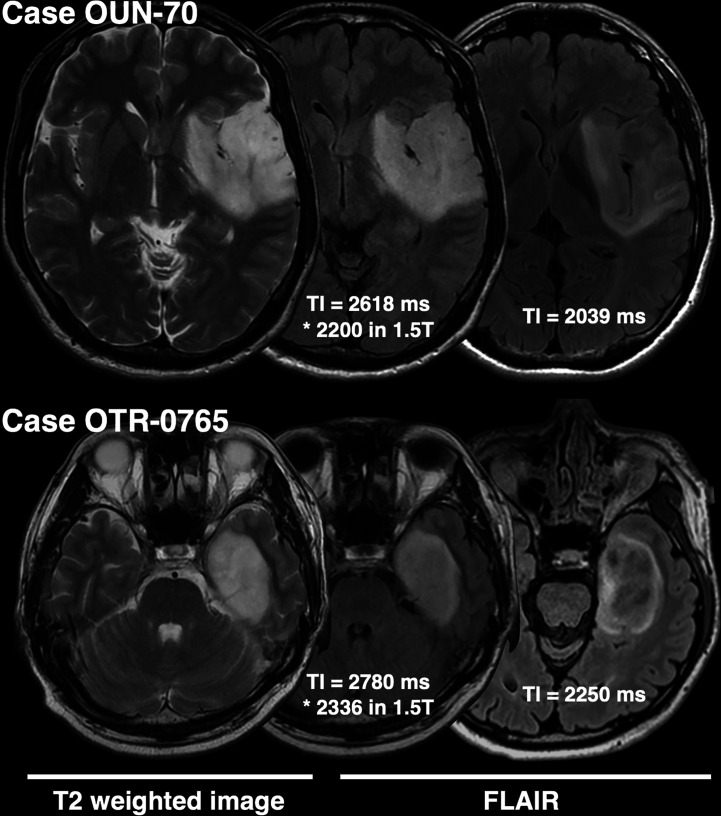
This figure shows two representative *IDH*mt, non-CODEL astrocytomas that had two FLAIR images scanned with different TIs. Both cases highlight the importance of TI for FLAIR acquisition in terms of visualization of the T2-FLAIR mismatch sign.
